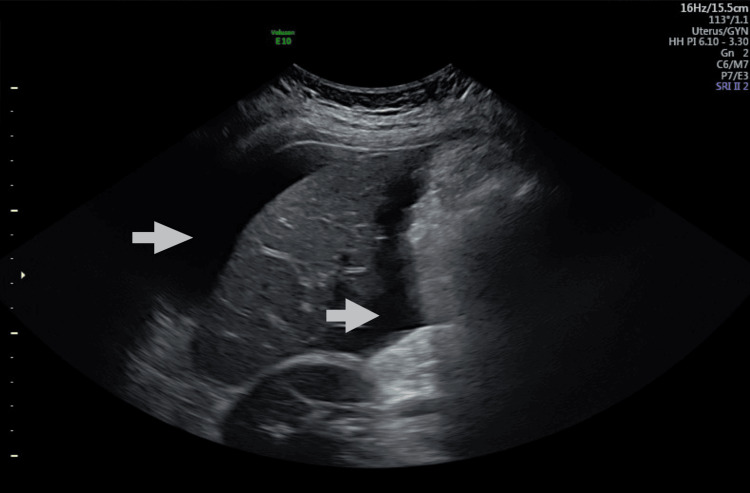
Ultrasound scan of the right upper abdomen Arrows showing fluid in the hepatorenal angle
